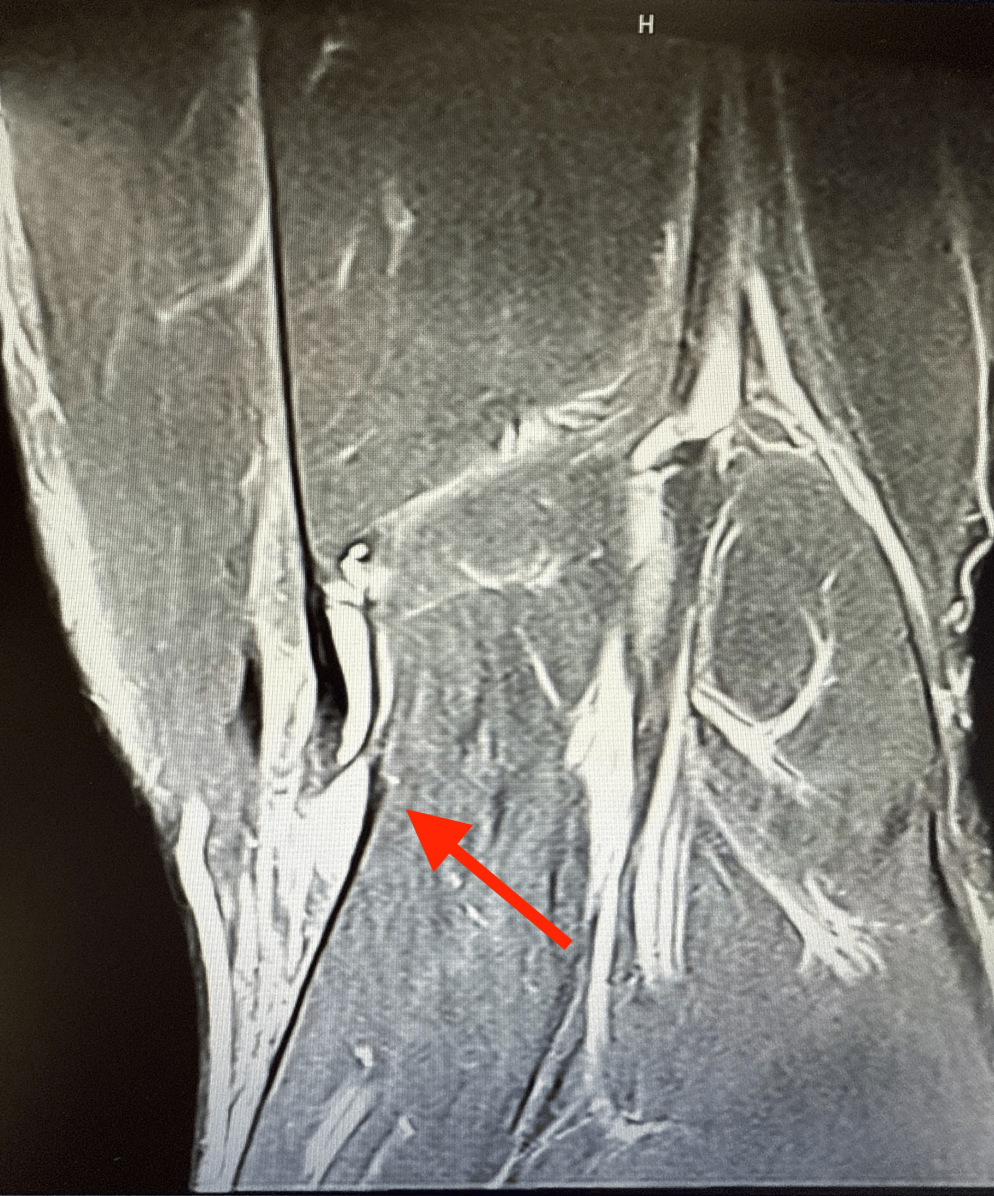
MRI of the knee showing discontinuity of the semitendinosus tendon with the surrounding fluid pocket (arrow).
